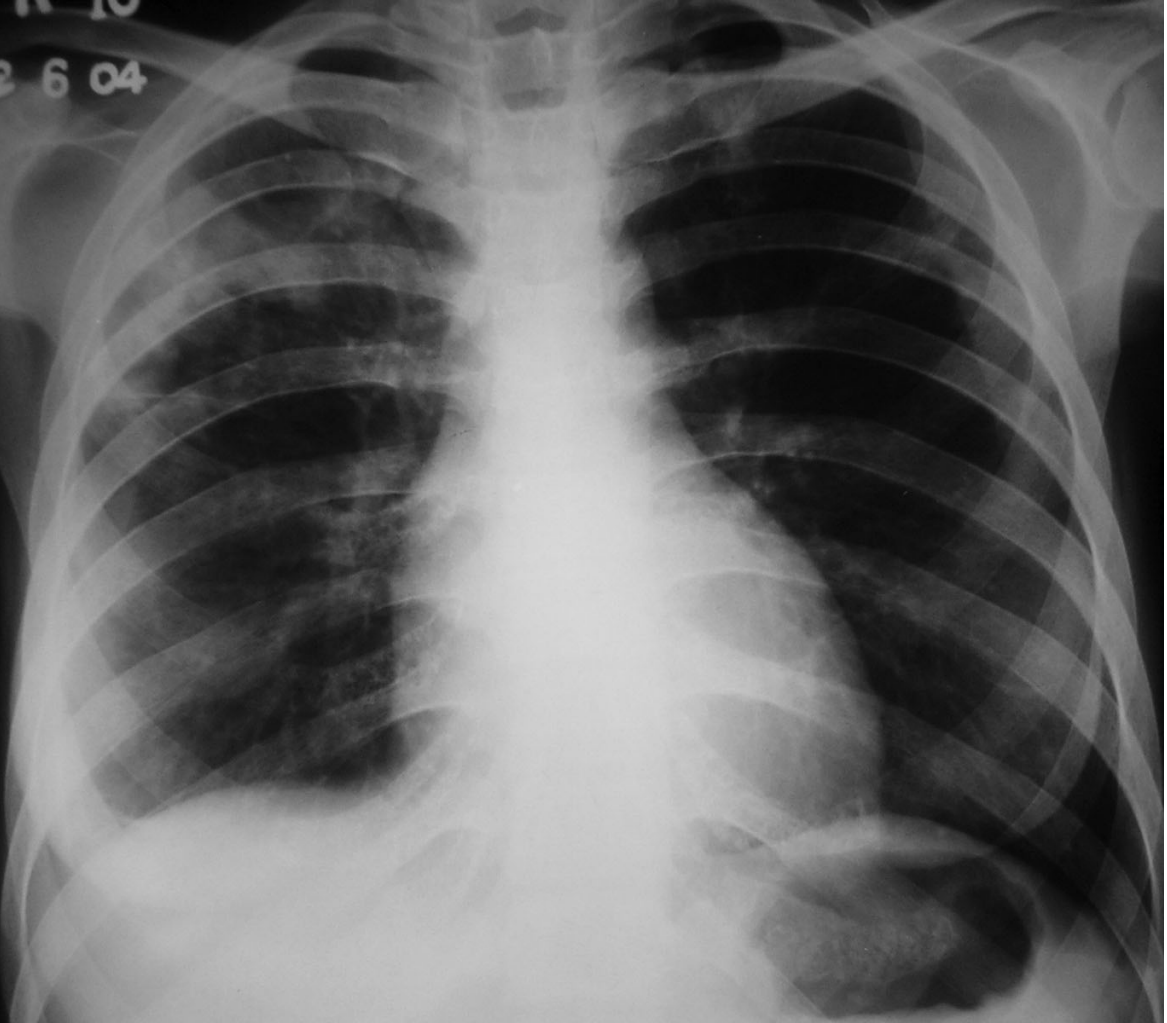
Chest radiograph showing right upper lobe consolidation (cardiac silhouette is normal).
